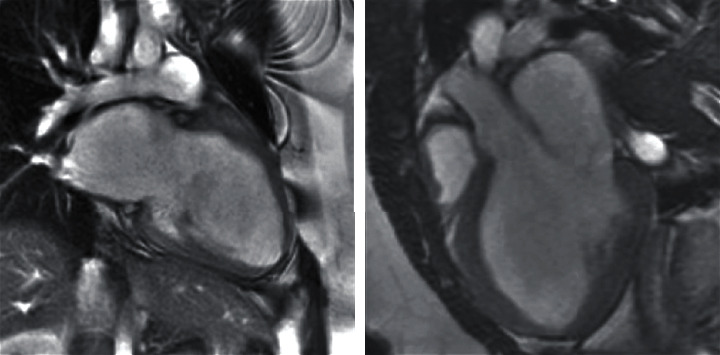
Cardiac magnetic resonance imaging video clips depicting severe left ventricular enlargement and markedly reduced left ventricular systolic function.
